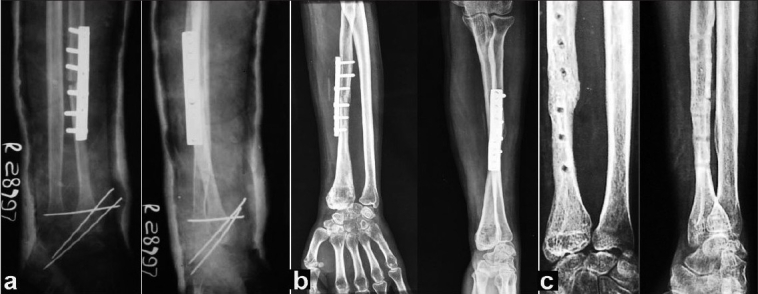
X-ray anteroposterior and lateral views of same patient (a) immediate postoperative X-Ray showing plate and K-wire in position. (b) 18 months postoperative X-Ray showing sound union at host graft junction. (c) 6 years postoperative X-Ray after plate removal showing union at host graft junction
